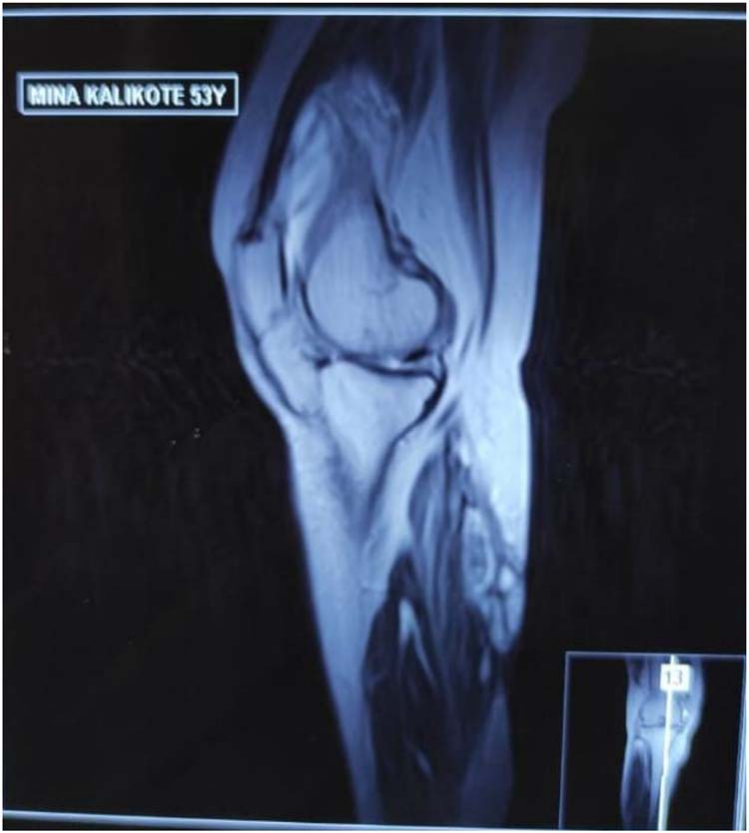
MRI of right calf muscle T2W image showing loculated collection over medial gastrocnemius muscle.
